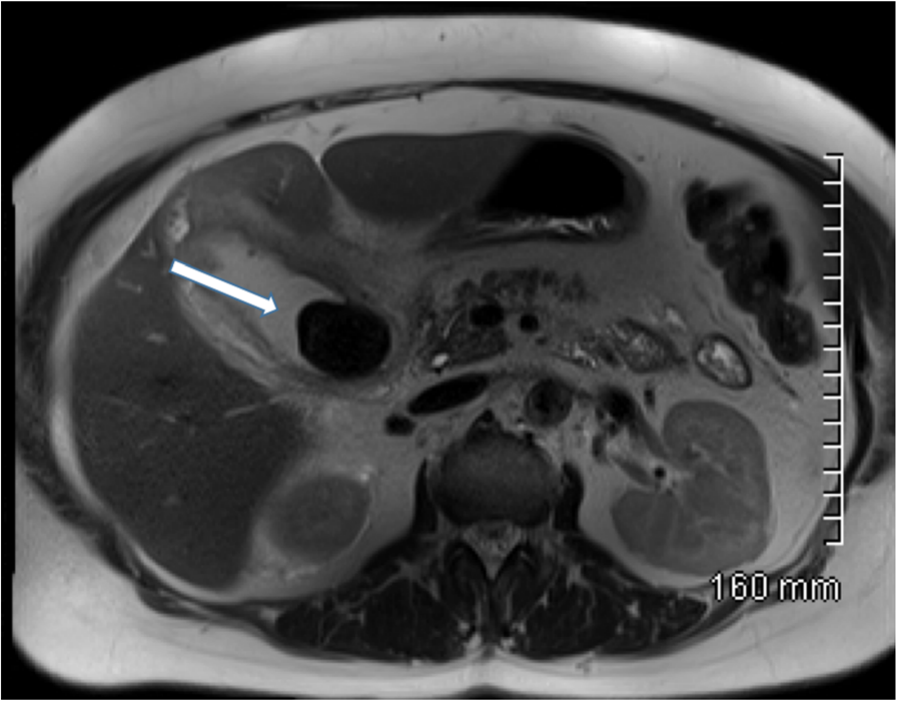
Axial T2-weighted MRI of the abdomen. There is a large gallstone within the gallbladder which is markedly hypointense relative to the surrounding hyperintense bile. The gallbladder wall is thickened with surrounding pericholecystic fluid consistent with acute cholecystitis
